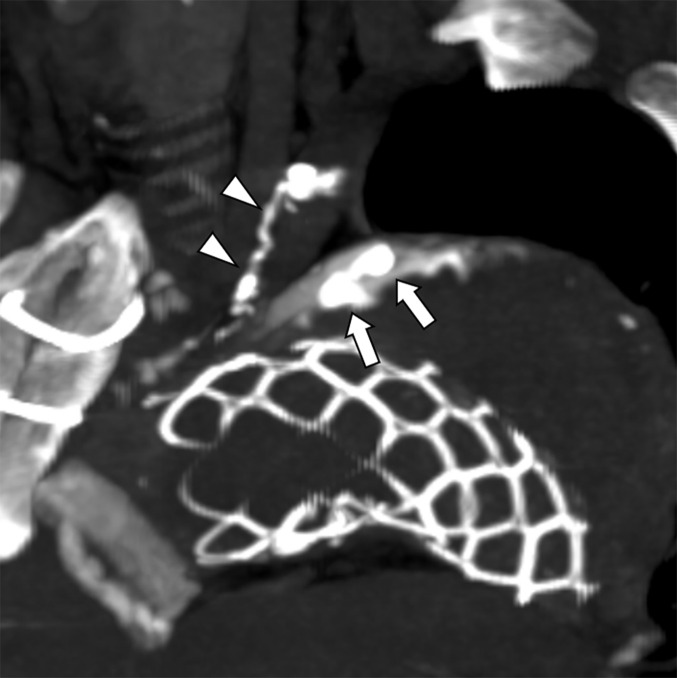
Non-contrast CT after TAE demonstrates deposition of lipiodol within the aneurysm sac (arrows) and the target artery (arrowheads). CT: computed tomography; TAE: transcatheter arterial embolization
